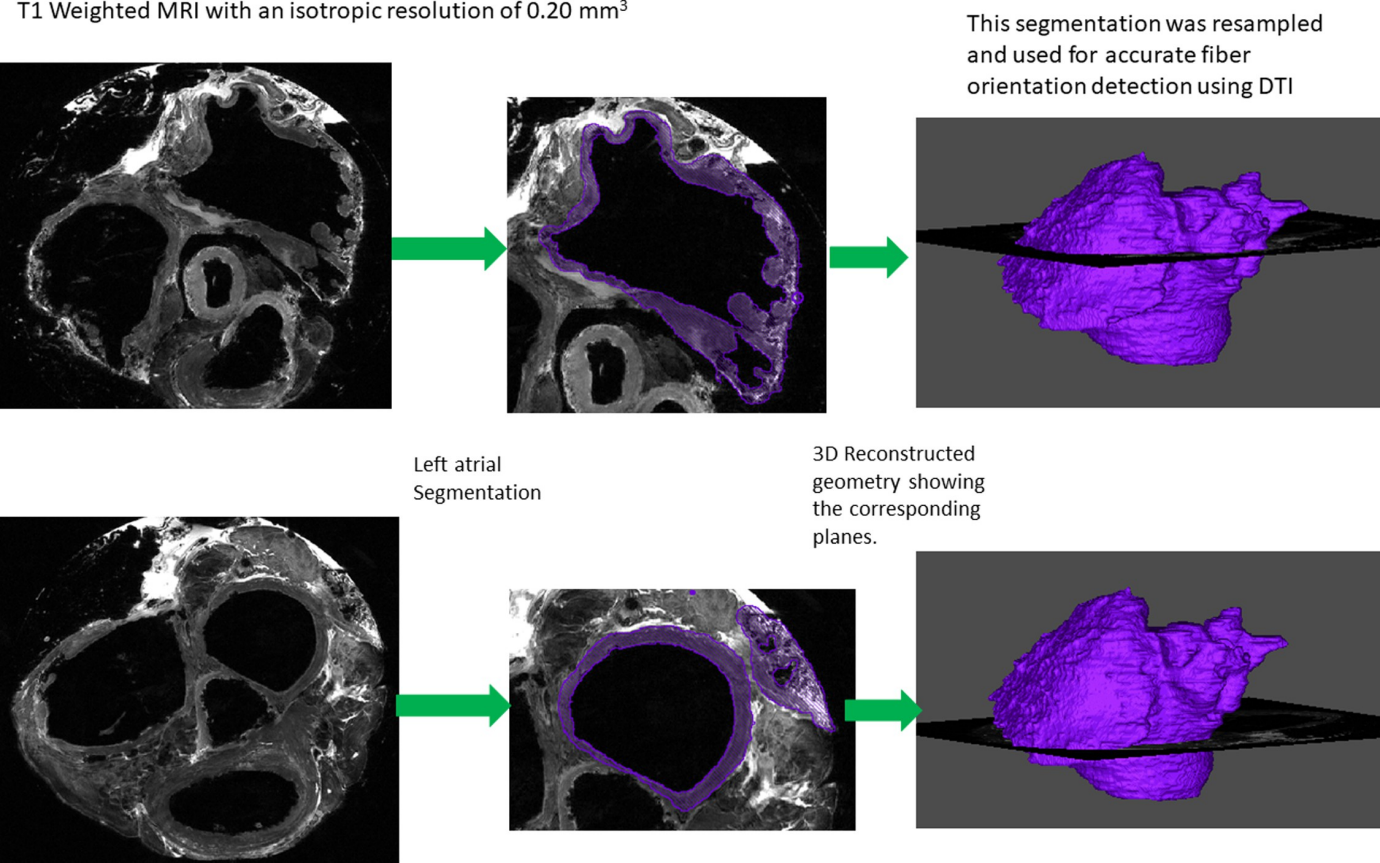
The segmentation of the high-resolution T1-weighted MRI used for the accurate fiber orientation detection using DTI.
